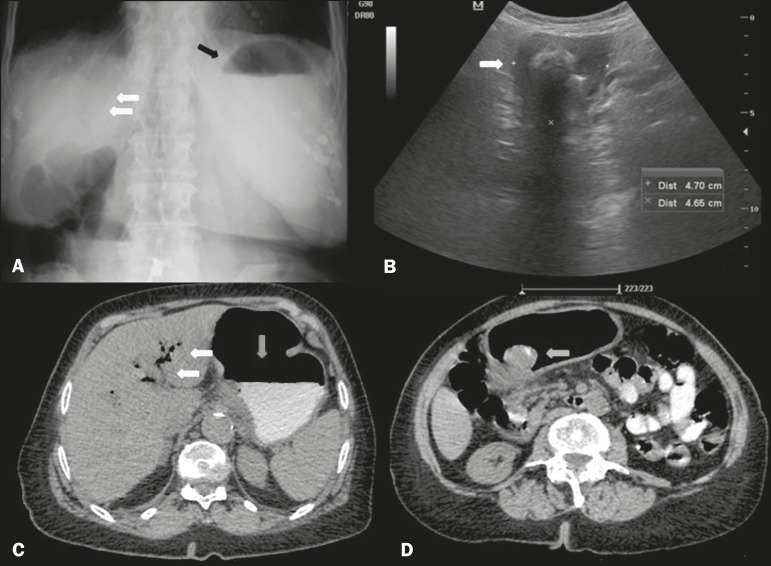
A: X-ray of the abdomen, with the patient standing, showing an
